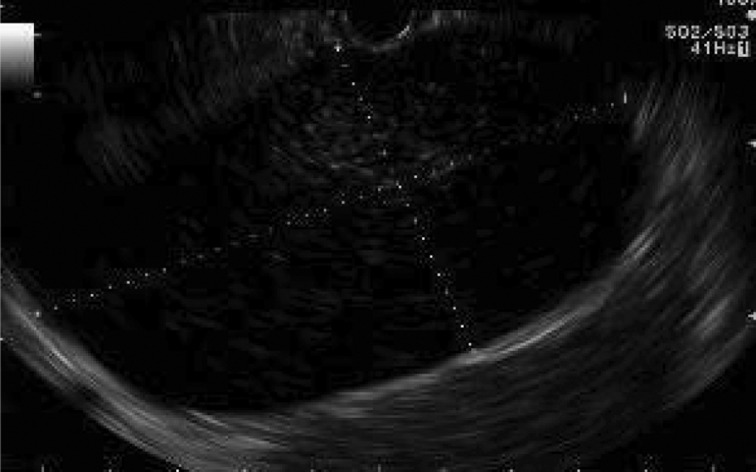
Endoscopic ultrasonography of a gastric duplication cyst
